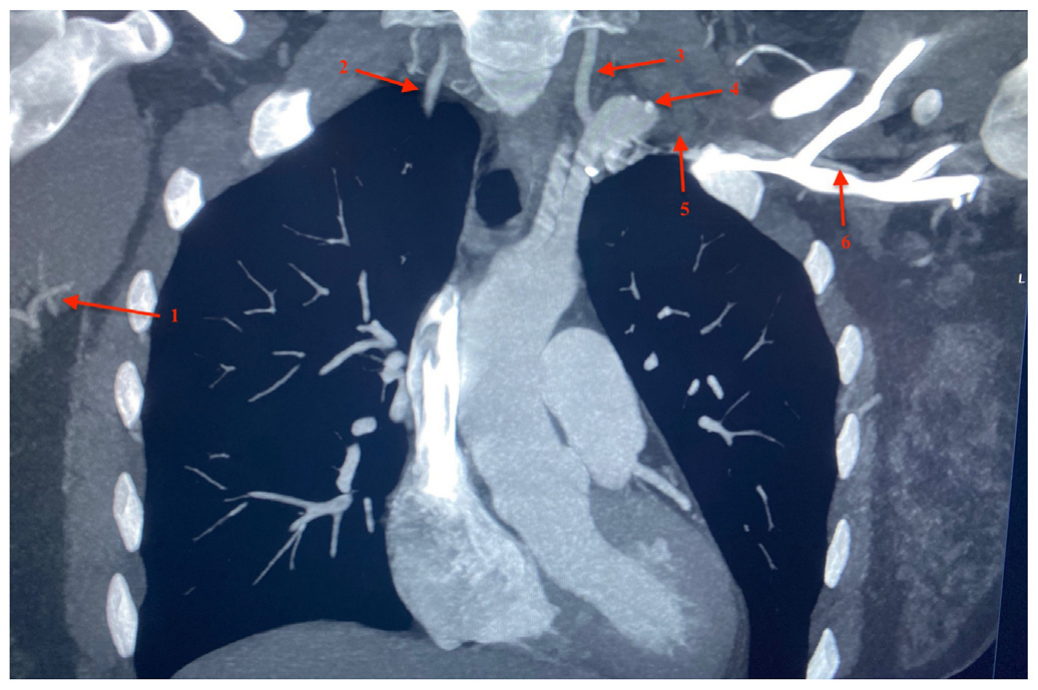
Computed tomography scan showing branches of distal right axillary artery (*1*), right vertebral artery (*2*), left vertebral artery (*3*), left subclavian artery aneurysm (*4*), expected course of left subclavian artery (*5*), and reconstitution of left axillary artery (*6*).
